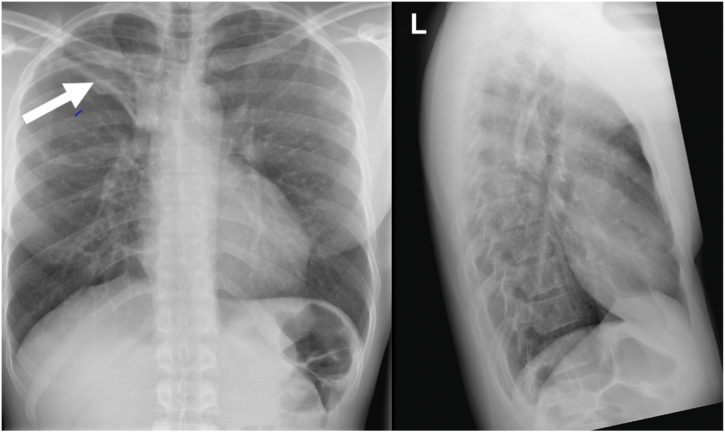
Chest X-ray (CXR) of a 33-year-old male from the Gambia with previous history of tuberculosis who presented with epigastric pain, weight loss and fatigue. The CXR shows consolidation in the upper zone of the right lung (white arrow).
